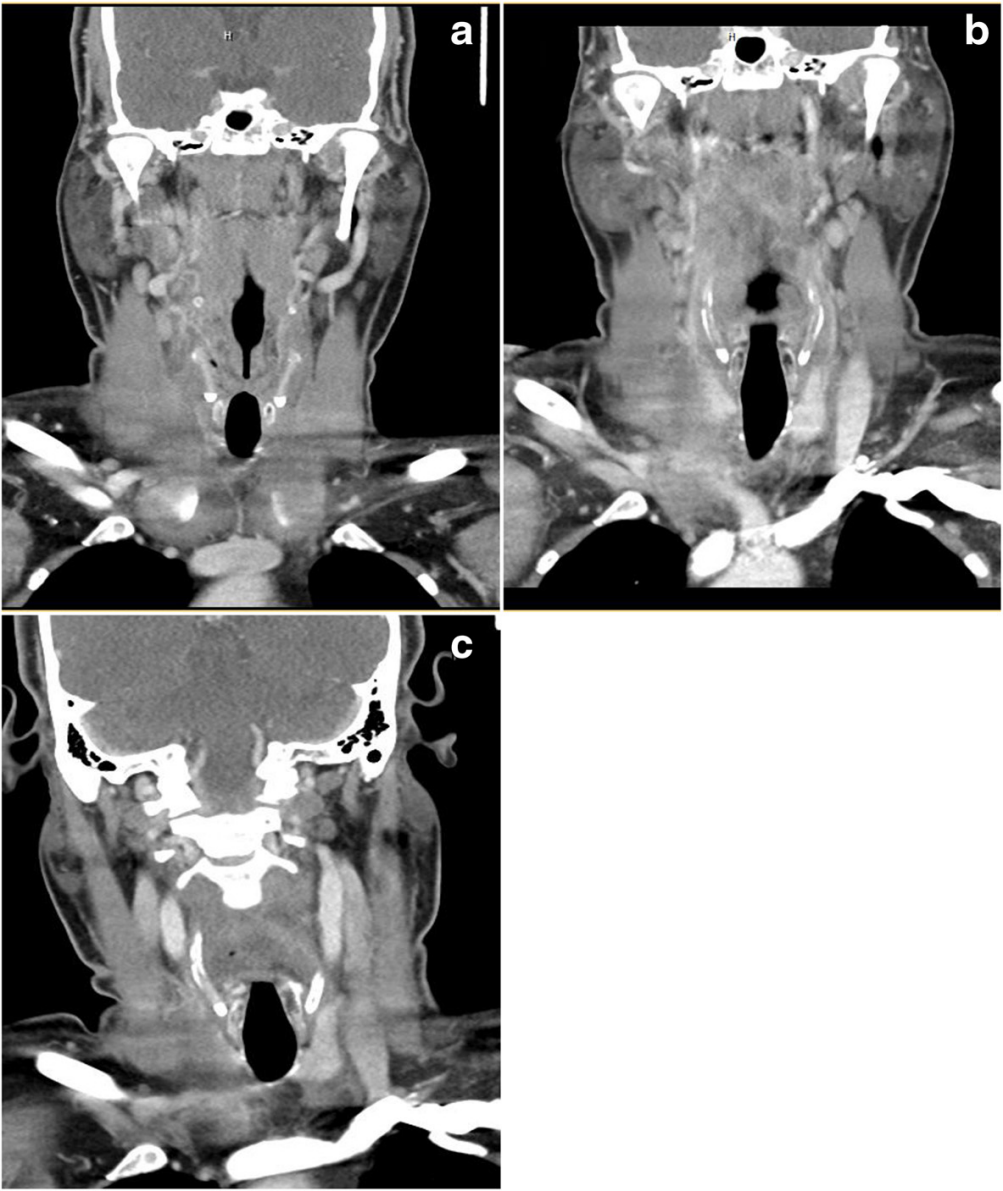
Neck CT Images. a Edema, stranding and exudates on initial presentation. b Worsening clinically after 4 days of antibiotic therapy. c Complete resolution 2 weeks after immunosuppressive therapy
